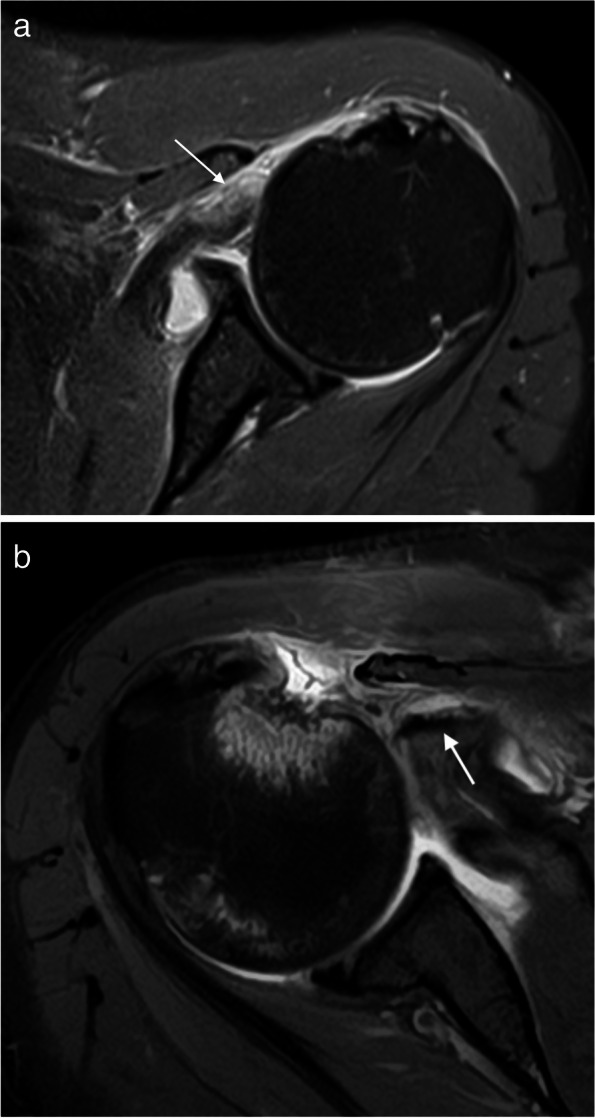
Traumatic subscapularis tears. a, b Axial fat-suppressed PD-weighted MRI images showing an acute SSC full thickness tear with retraction of the tendon (arrow in a) and an acute bony avulsion of the insertion of SSC tendon in the lesser tuberosity (arrow)
